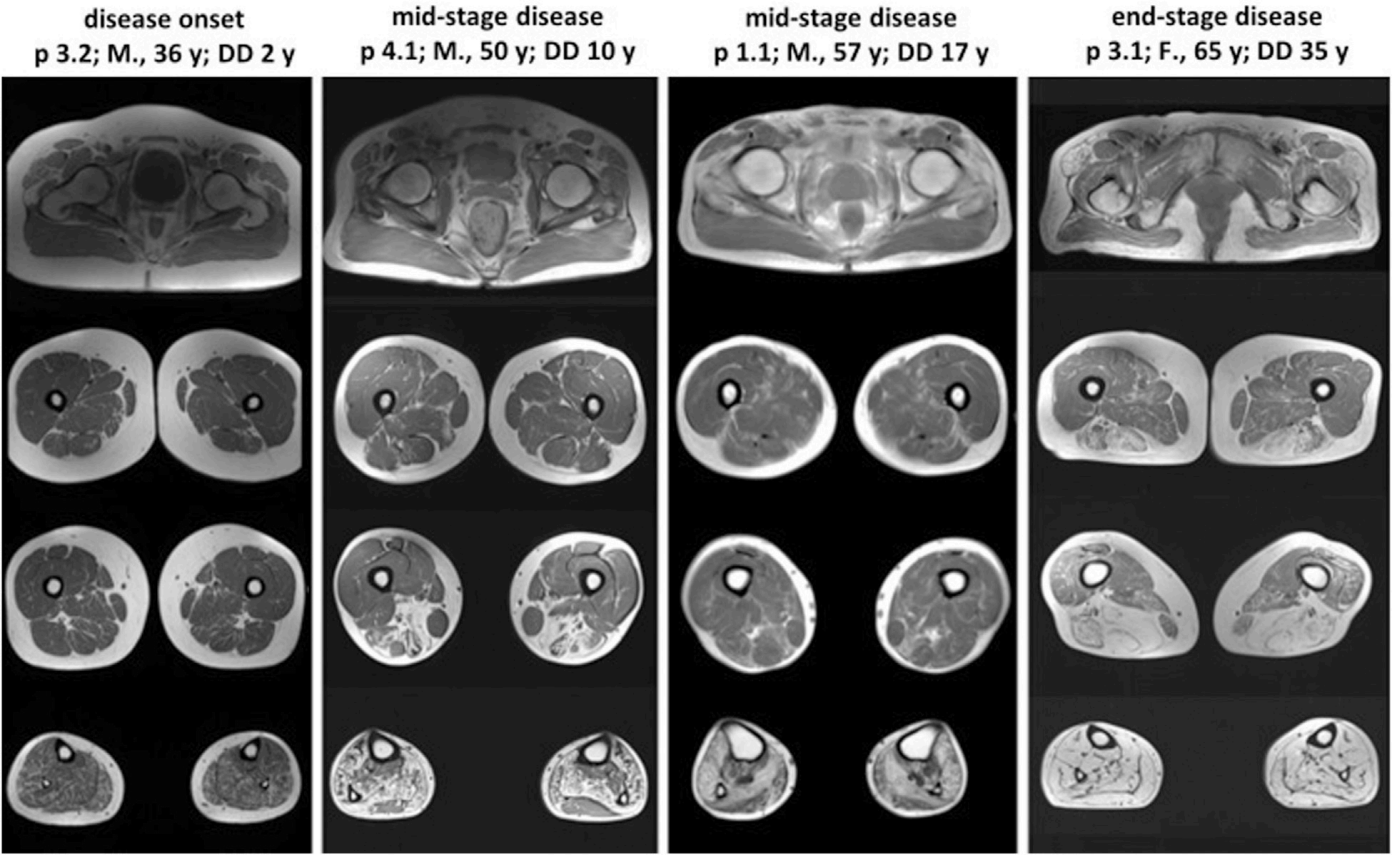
Lower limb muscle MRI in patients with different stages of VCPDM. The characteristic pattern of muscle involvement was observed in patients with the mid-stage of the disease. T1-weighted MRI revealed the typical involvement of lower leg muscles, with relatively spared tibialis posterior and lateral gastrocnemius. The patient with disease onset demonstrated mild diffusely affected posterior compartment of lower leg muscles. In the patient with end-stage disease, complete fatty replacement of lower leg muscles was noted. The most pronounced affected thigh muscles were the long head of the biceps femoris and the semimembranosus with spared gracilis in all patients. M, male; F, female; y, years; p, patient; DD, disease duration.
